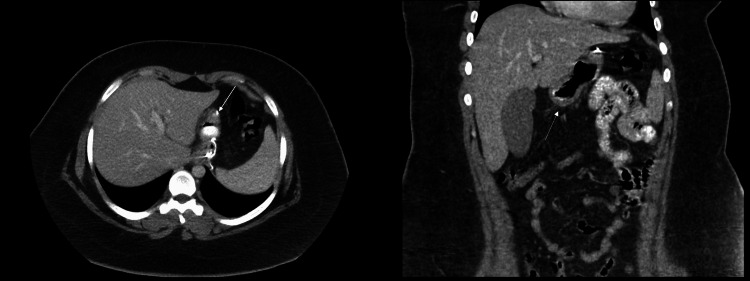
CT of the abdomen and pelvis with intravenous and oral contrast (axial and coronal views) Arrows show a healthy sleeve stomach with an intact staple line, without evidence of any extraluminal leak of oral contrast. No other pathology was noted; there was normal enhancement of the gastric tissue.
